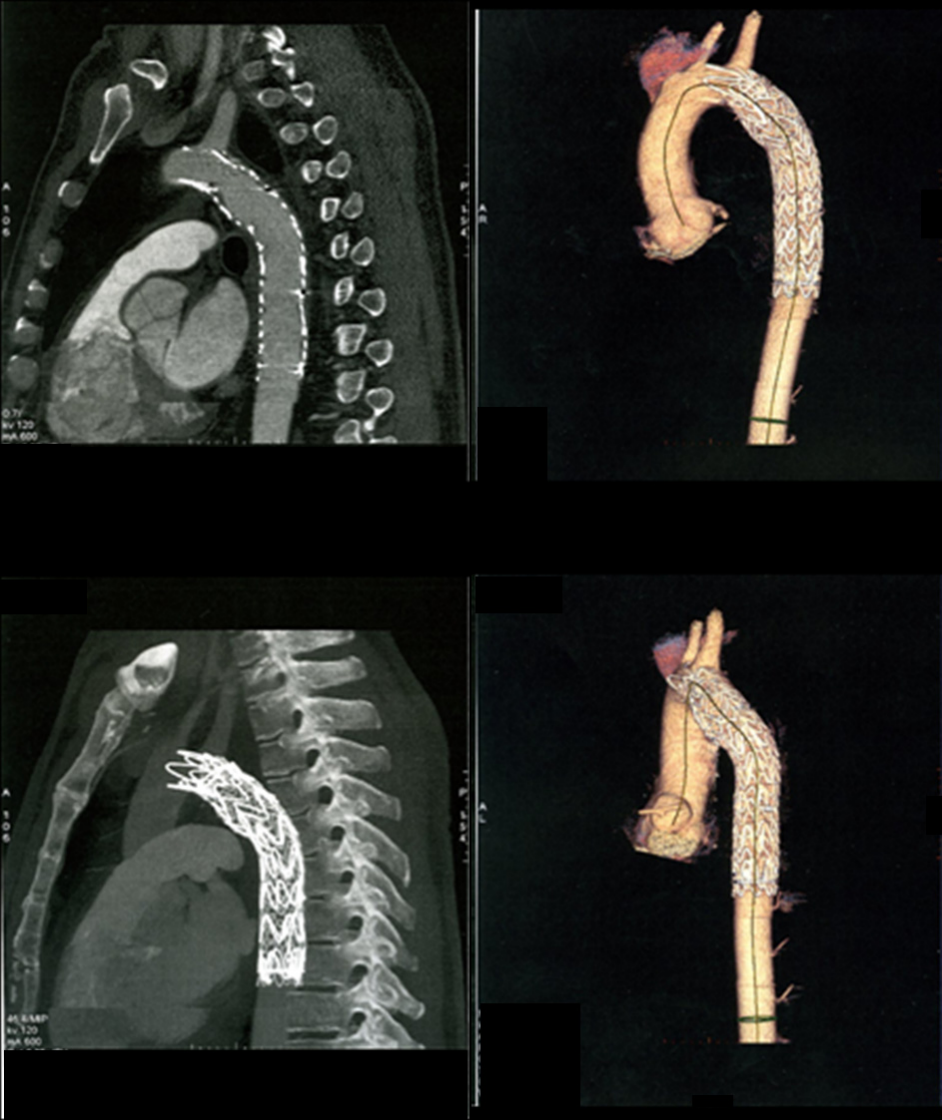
CT scan at 18 months. Regular diameter of the graft, normal renal perfusion, no signs of any endoleaks.
